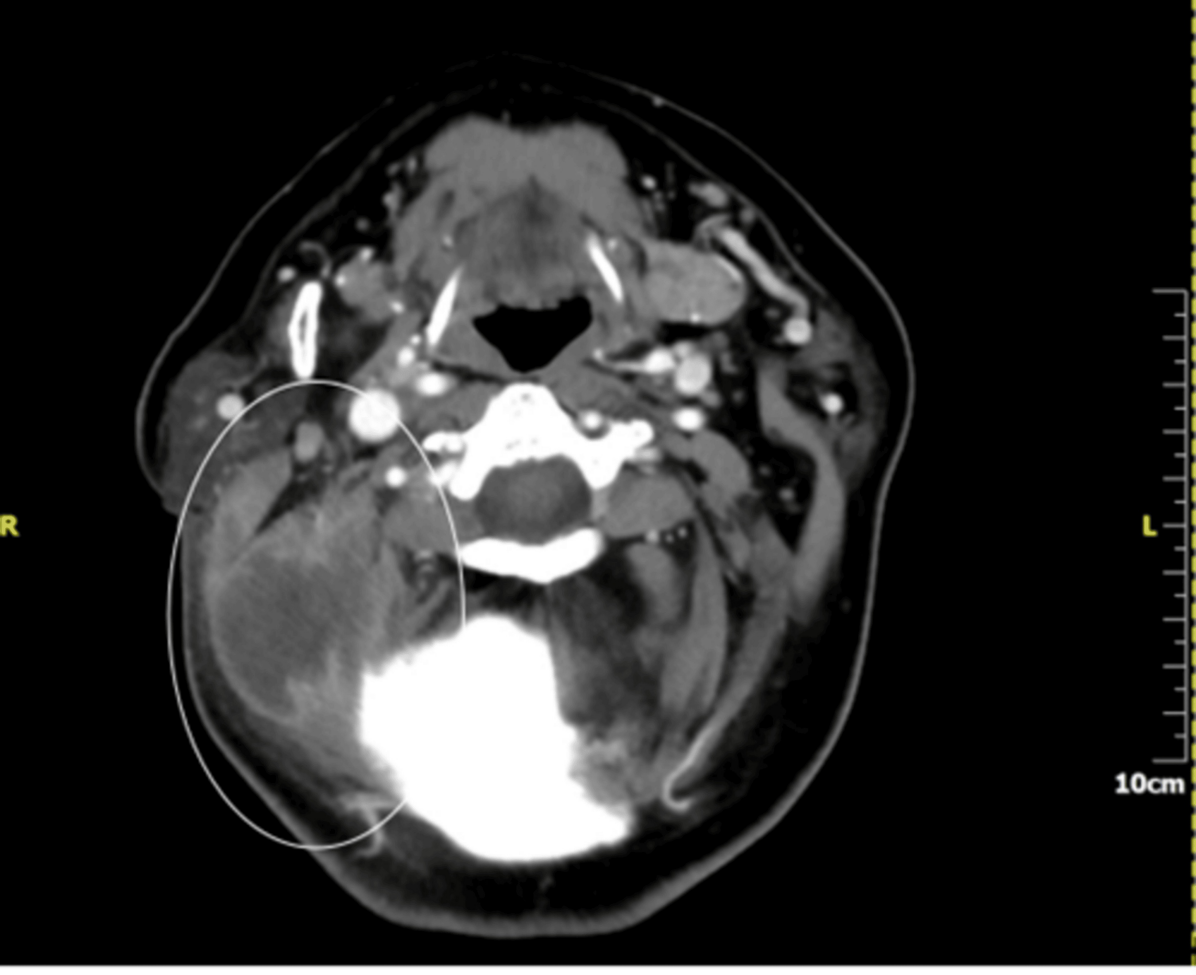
Axial plane of a CT scan of the neck with contrast showing a well-demarcated fluid collection with a peripheral rim enhancement indicating highly an abscess deep to the sternocleidomastoid muscle (highlighted with the white oval shape).
